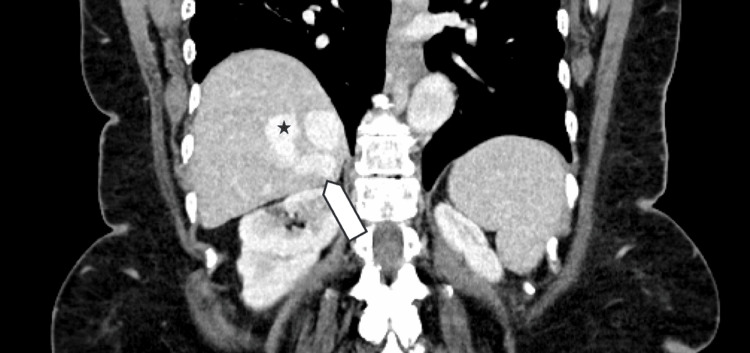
Portal venous-phase coronal CT showing a portosystemic shunt The arrow indicates the feeding right portal vein, and the star marks the draining right hepatic vein.
